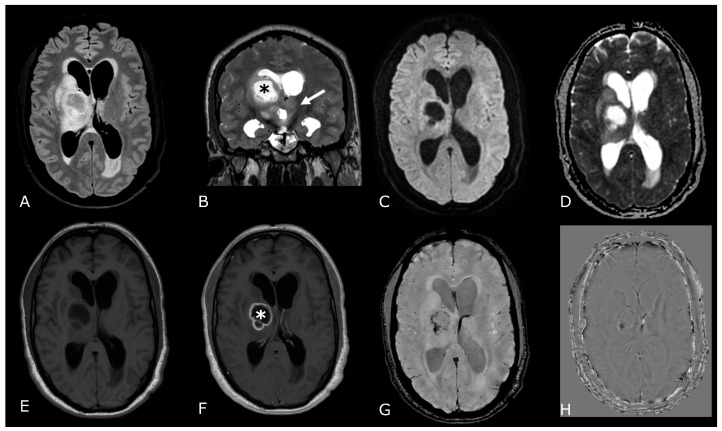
Conventional MR sequences: necrosis and hemorrhages. Right deep thalamo-capsular IDH-wt GB. The lesion shows a necrotic core (asterisk in (B,F)) and a thick and irregular rim of hypercellular tissue with restricted diffusion (C,D) and contrast-enhancement ((F) compared to (E)). (G,H) demonstrate hemosiderin marginal deposits (hypointense on both SWI and phase-map respectively) suggestive of intratumoral bleedings. The “rim enhancing” lesion is surrounded by a peripheral heterogeneous area of abnormal T2w/FLAIR signal (A,B), reflecting infiltrative “non enhancing” tumor and vasogenic edema that also involves the mesial surface of the contralateral thalamus and hypothalamus (arrow in (B). The caudal extension determines stenosis of the Sylvian aqueduct and consequently supratentorial hydrocephalus.
